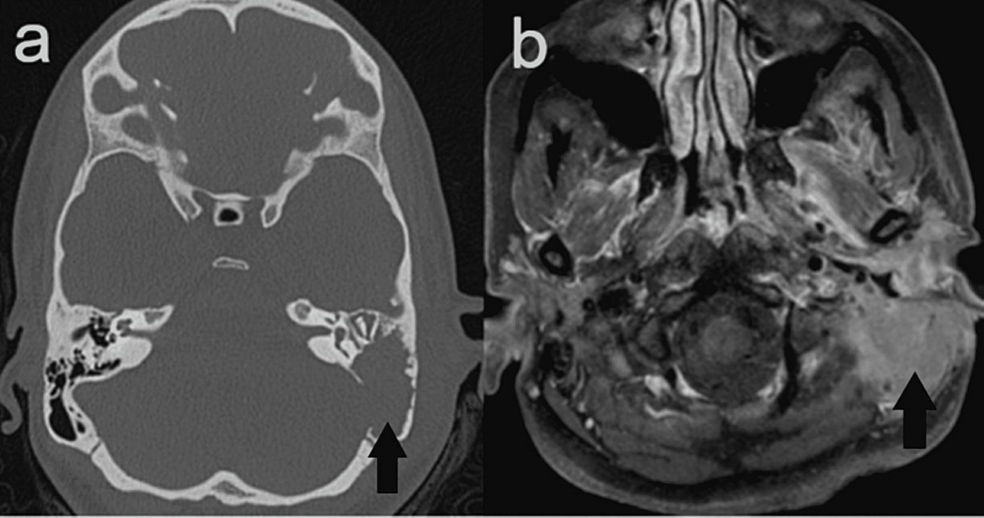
CT of the temporal bone (A) and MRI of the brain (B) A. Axial view of CT scan showing coalescent process involving the left temporal bone. B. Axial view of T1-weighted MRI demonstrating a solidly enhancing, mass-like lesion centered within the mastoid segment of left temporal bone, with associated osseous destruction, extending into the left periauricular soft tissues, left external auditory canal, and minimally into the epidural space along the inferior aspect of the left temporal lobe.
